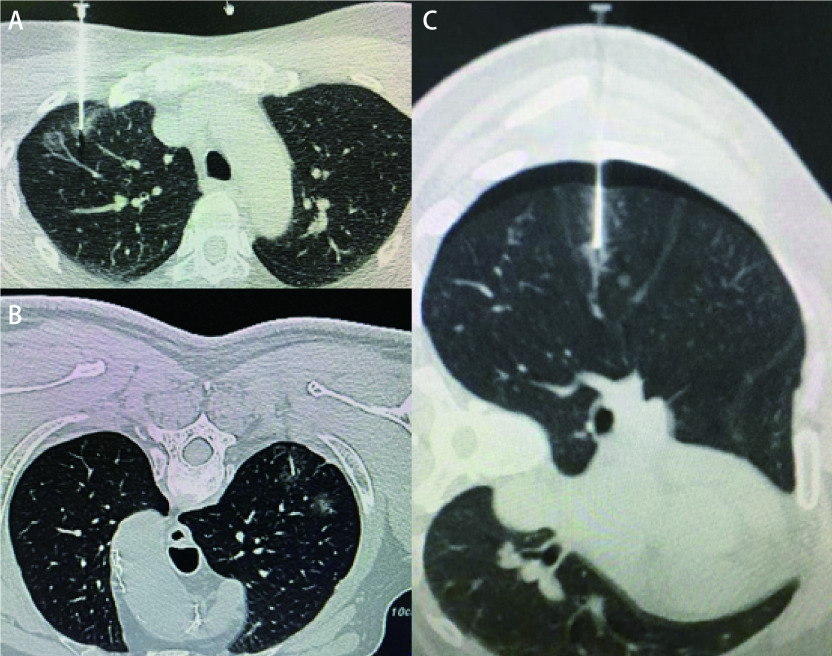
CT引导下医用胶在胸腔镜术前肺结节定位。A：依据“垂直就近”的原则定位；B：最佳穿刺路径被肩胛骨或肋骨遮挡，医用胶也可准确定位；C：发生气胸时，定位中叶结节。 The application of CT-guided localization with medical glue for small pulmonary nodules before VATS. A: Localization according to the principle of "vertical proximity"; B: The medical glue can be accurately localizated, although the best puncture path is blocked by shoulder blades or ribs. C: When pneumothorax occurs, locate the middle lobe nodule. CT: computed tomography; VATS: video-assisted thoracic surgery.
